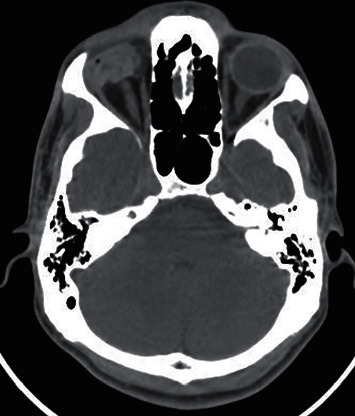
Open globe injury in a 26-year-old man. Axial unenhanced CT image showing right disorganized globe.
